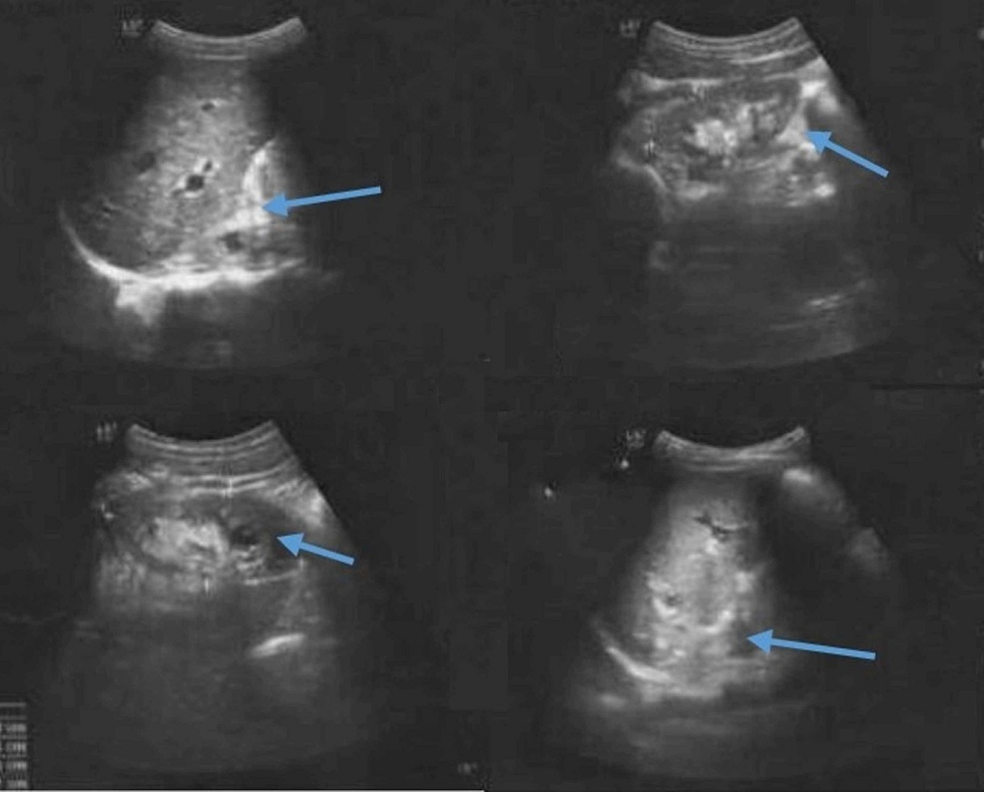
Ultrasound abdomen showing heterogeneous echotexture, cystic formation, and pulsation vascular center in the left kidney (blue arrows).
